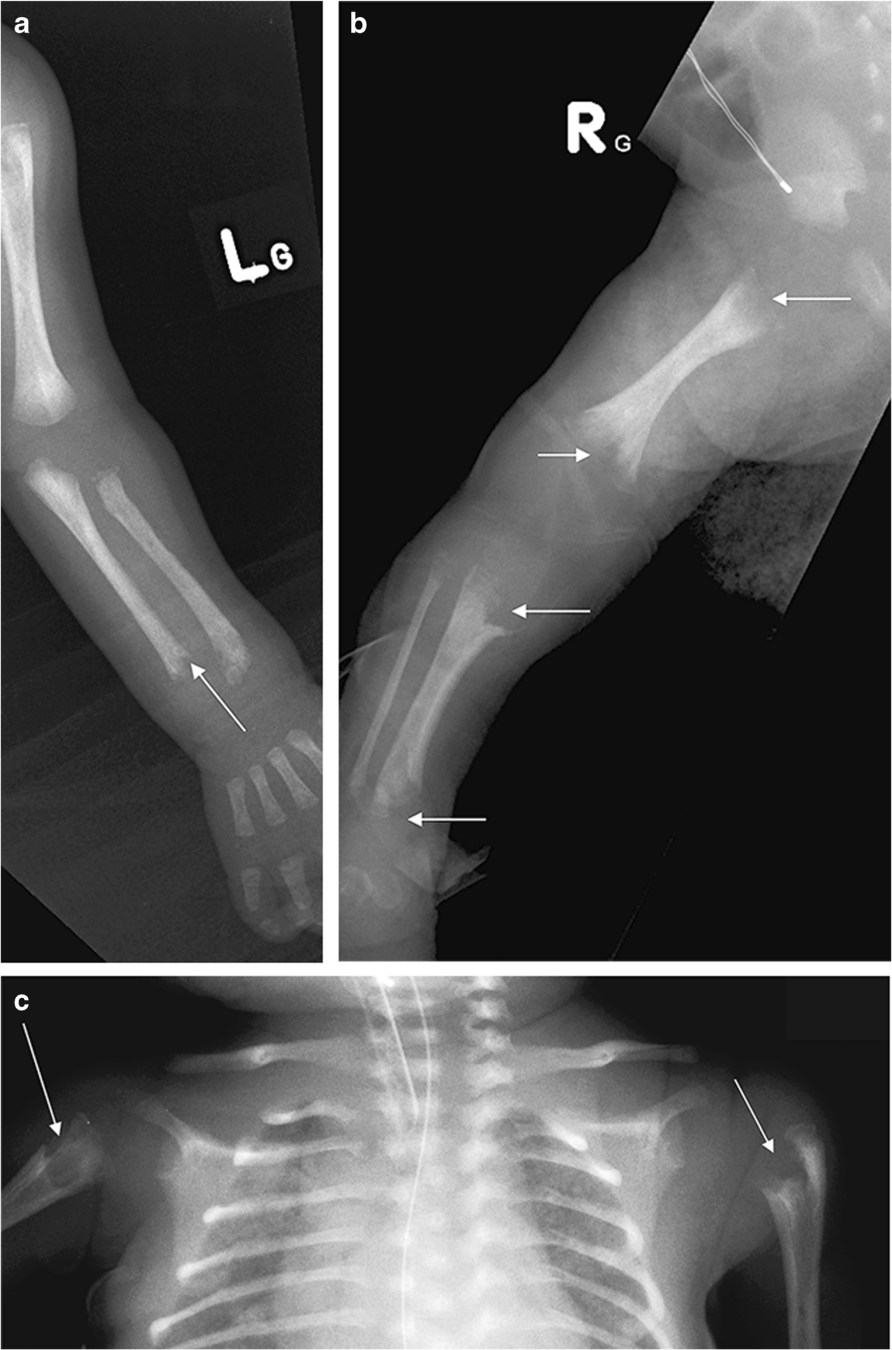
Imaging features of hypophosphatasia in a 3-week-old girl who died with HPP who died within the first 3 months of life*.*a-c Anteroposterior radiographs show metaphyseal “tongues” of radiolucency (*arrow*) in the left upper limb (a), wide irregular metaphyses (*arrows*) and absent ossification of epiphyses of the knee in the right lower limb (b), and slender ribs and metaphyseal “tongues” of radiolucency (*arrows*) in the upper chest (c)
